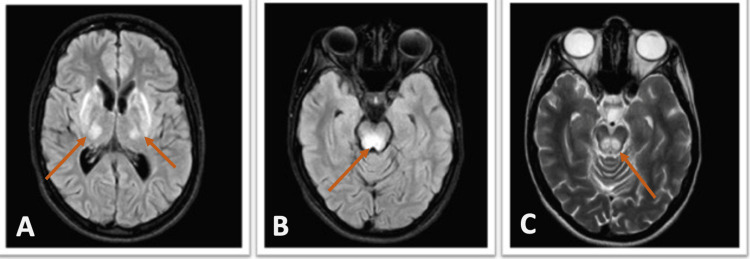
Patient's brain MRI. (A) T2 FLAIR images revealed symmetrical hypersignal in the thalami, lentiform nuclei, and lateral aspects of the brainstem. (B, C) Axial T2 FLAIR images showed hypersignal in the tegmental and mesencephalic roof.
